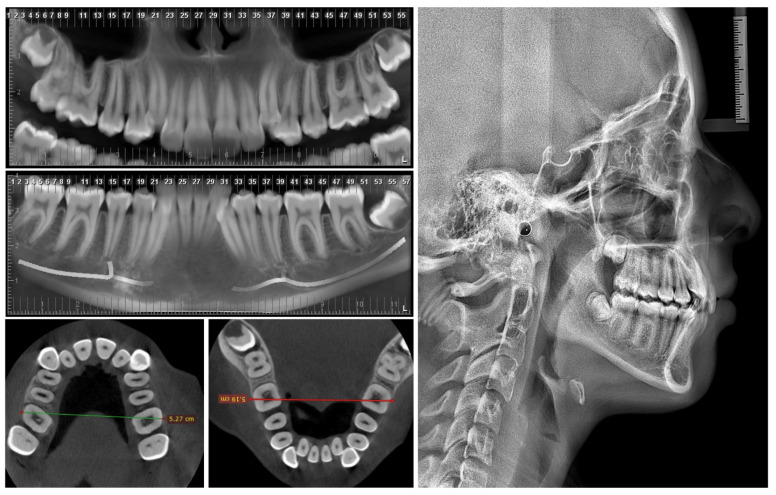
Diagnostic radiographs and initial CBCT scans.
